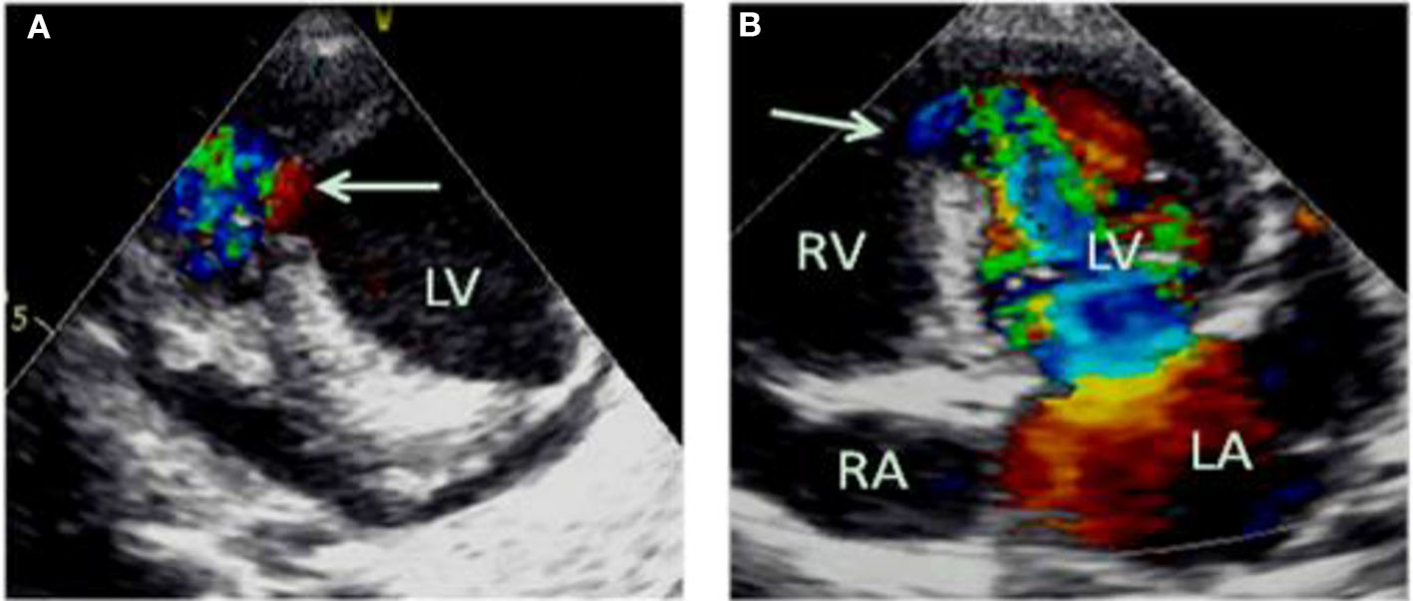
Emergency bedside transthoracic echocardiography revealed funnel shaped M-VSD (A). The white arrows indicate the left and right ventricular shunt orifice (A,B). Color Doppler flow imaging show a bidirectional shunt between the left and right ventricles (A,B). LA, left atrium; RA, right atrium; LV, left ventricle; RV, right ventricle.
